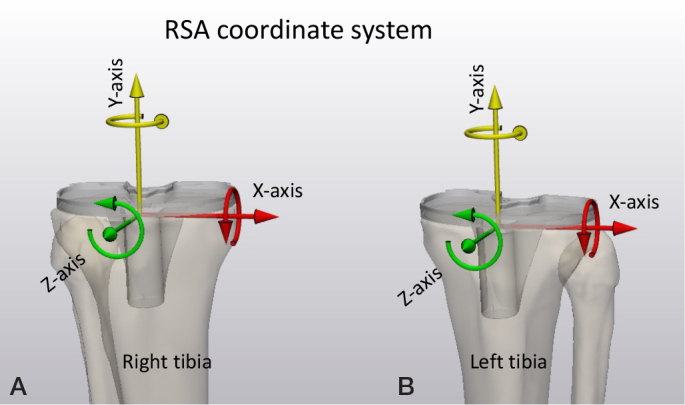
The RSA migration coordinate system for a right-sided (A) and a left-sided (B) tibia. For left-sided tibias, translations in the X-axis direction, and rotations about the Y-axis and Z-axis must be multiplied by minus one to convert them to right-side tibias. For the CT coordinate system (shown in Figure 3B), translations in the X-axis direction, and rotations about the Y-axis and Z-axis must be multiplied by minus one to convert them to right-sided tibias.
